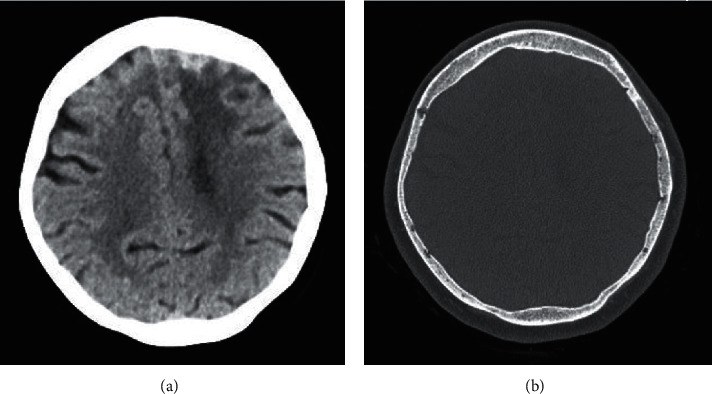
CT head: (a) left frontal extra-axial lesion with patchy calcification and vasogenic oedema; (b) CT bone window setting showing osteopenia but no bony erosion or hyperostosis.
